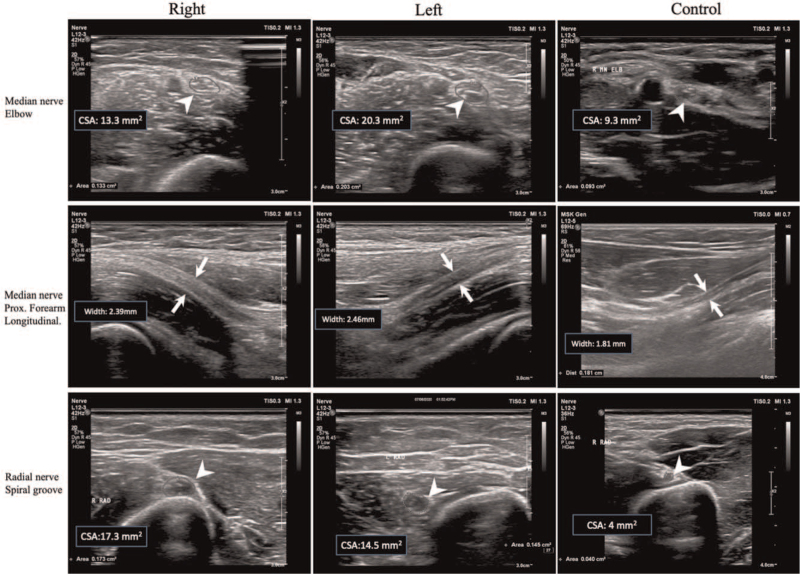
Bilateral swelling in the bilateral median and radial nerves in case 1. Ultrasonography showing enlargement of the bilateral median nerves in the elbow region and the bilateral radial nerves in the spiral groove region. Swelling was not observed in the control. CSA = cross-sectional area.
